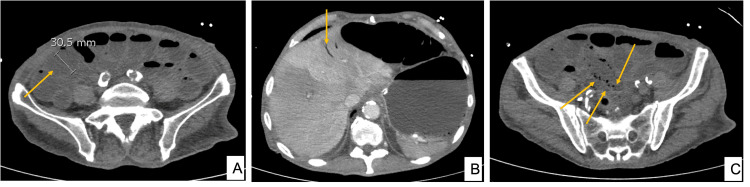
CT of patient one The images demonstrate dilated small bowel loops (A), with pneumatosis intestinalis (B), along with portal vein gas (C) CT: computed tomography
